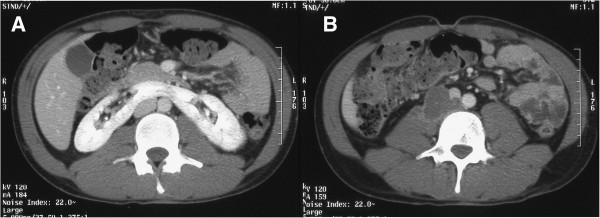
Retroperitoneal right laterocaval mass. CT shows the coexistence of a horseshoe kidney (A) and a retroperitoneal right laterocaval mass of the maximum diameter of 7 × 9 cm, indissociable from the iliopsoas muscle and without clear signs of infiltration of the caval wall (B).
